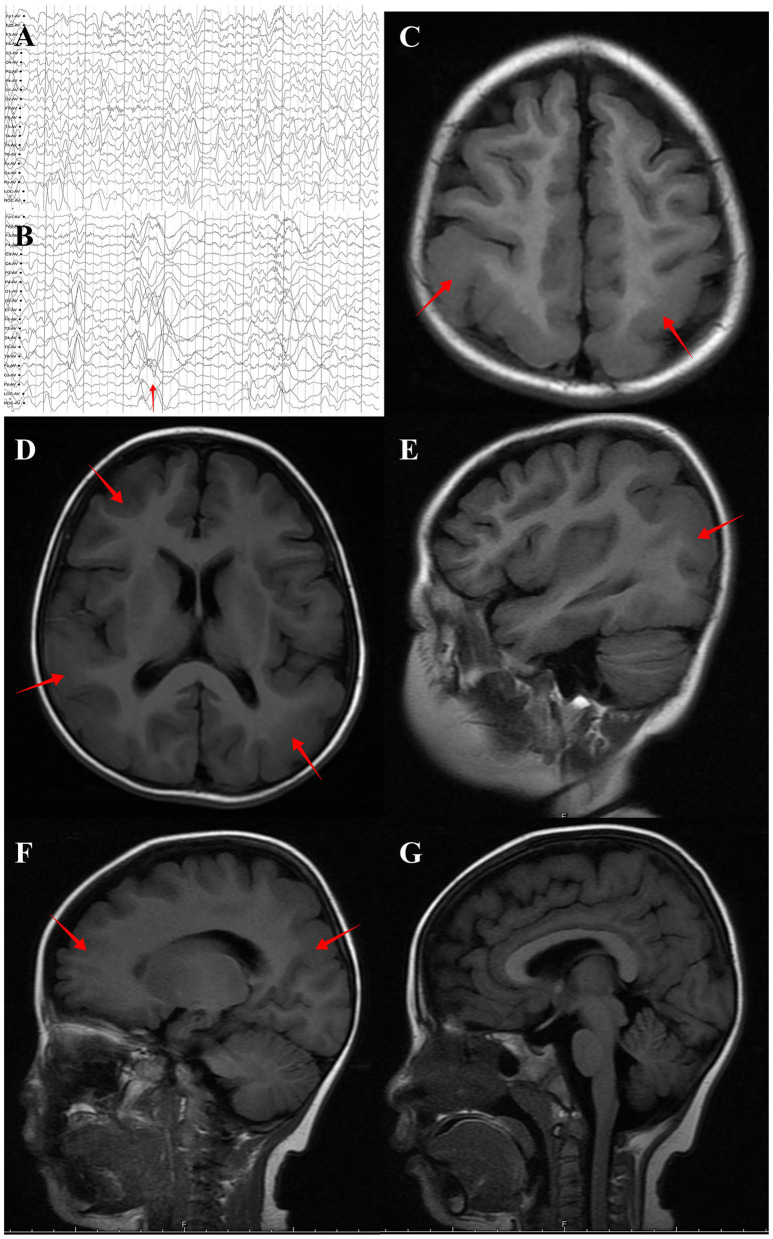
Brain MRI and EEG of the individual with *DYNC1H1* variant p.Arg292Trp. (A) Interictal EEG showed multifocal spike-wave complexes at 2 years old. (B) EEG at spasm onset (2 years old) revealed brief, high γ oscillations combined with slow delta waves (red arrow). (C) (Axial plane, T1 flair), (D) (Axial plane, T1 flair), (E) (Sagittal plane, T1 flair), (F) (Sagittal plane, T1 flair) and (G) (Sagittal plane, midline, T1 flair). MRI performed at 4 years old and revealed posterior predominant pachygyria (red arrow).
